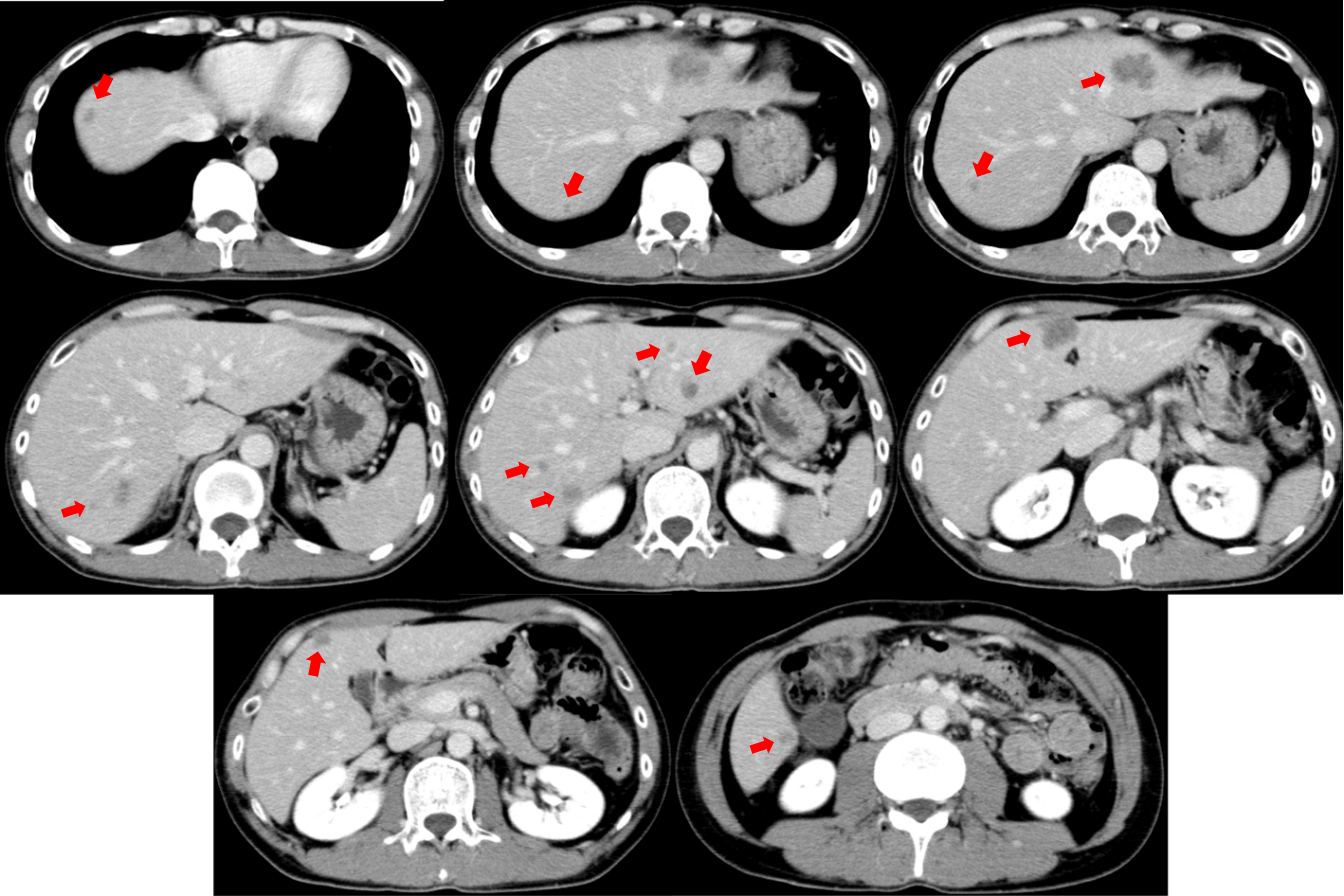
Contrast-enhanced computed tomographic image 1 month after the first operation. The number of liver metastases increased from 2 to 12 lesions
